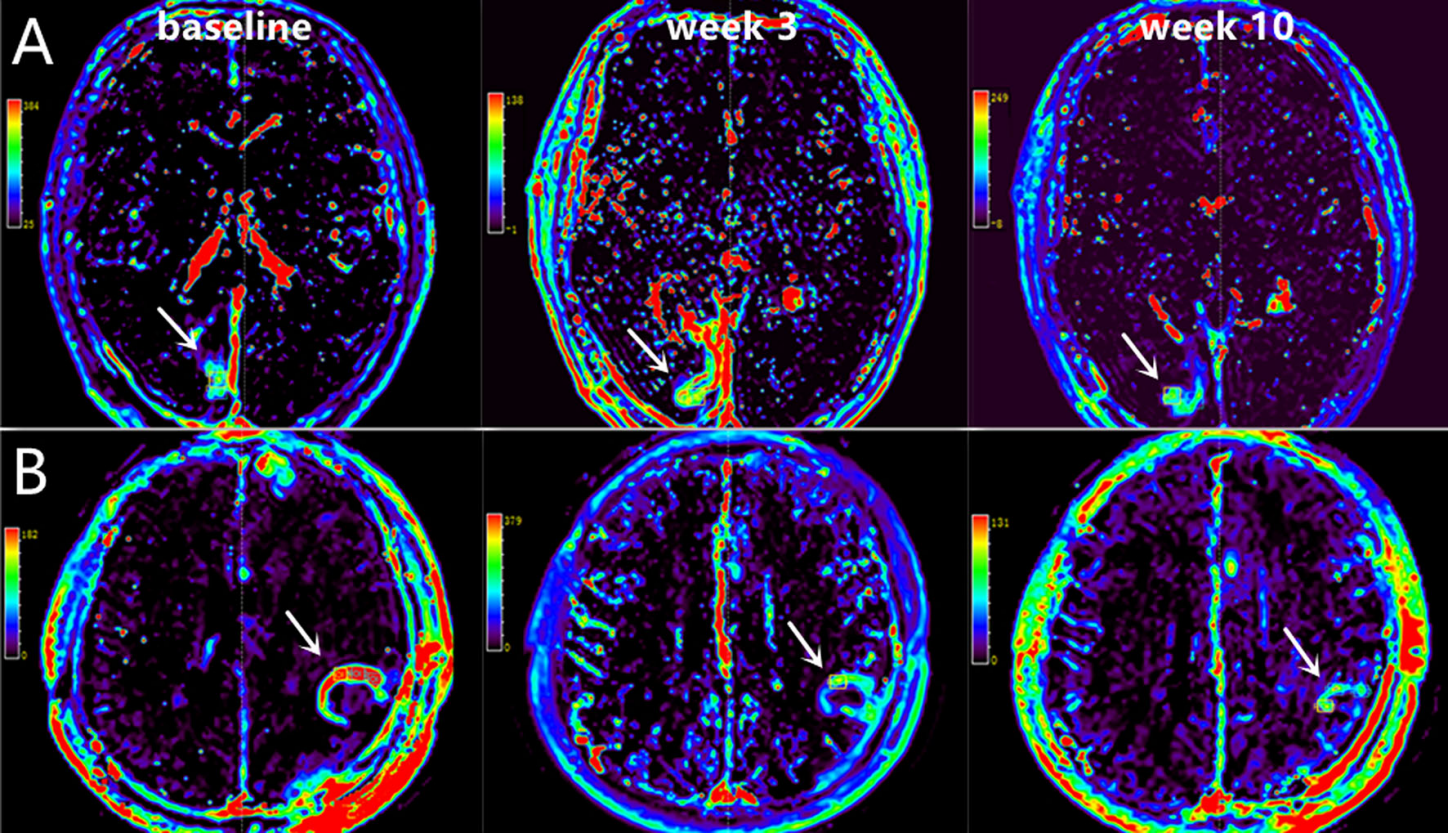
Representative DCE-MRI scans at baseline, week 3 and week 10 in two patients with OS of 41.92 months [A, Male, 53 years old, decrease in K^trans^=13.12 (min^-1^)] and 22.18 months [B, Male, 48 years old, decrease in K^trans^=-139.82 (min^-1^)].
